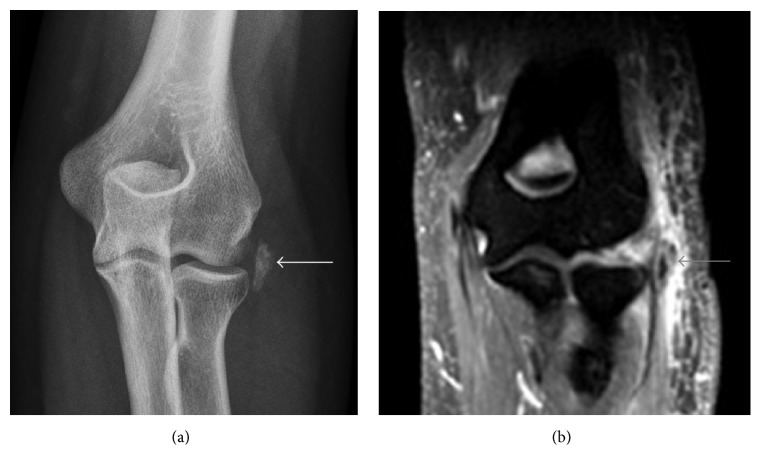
Calcific tendinitis of the common extensor tendon origin. (a) AP radiograph shows amorphous calcifications along the lateral aspect of the elbow joint (white arrow). (b) Exuberant soft tissue edema can be seen around the calcifications, which are centered within the common extensor tendon (grey arrow).
